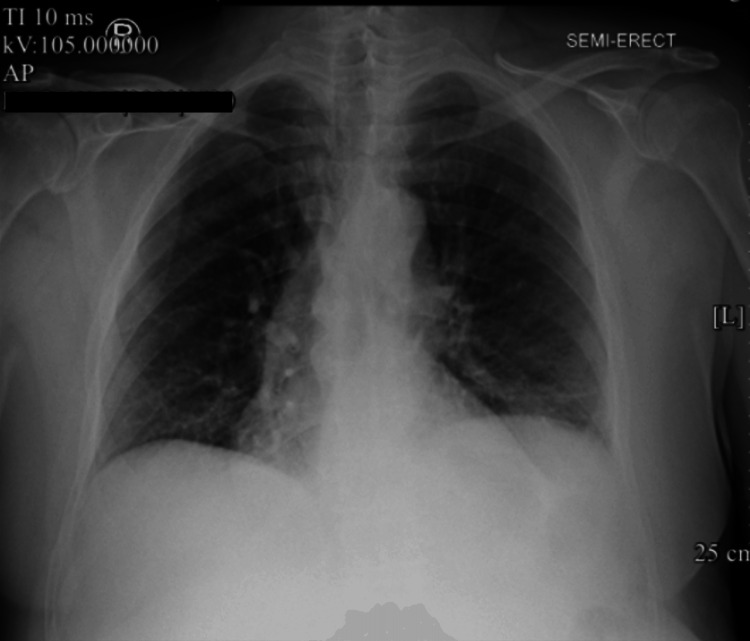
Chest X-ray on admission showing no focal pulmonary consolidations or pleural effusions.
